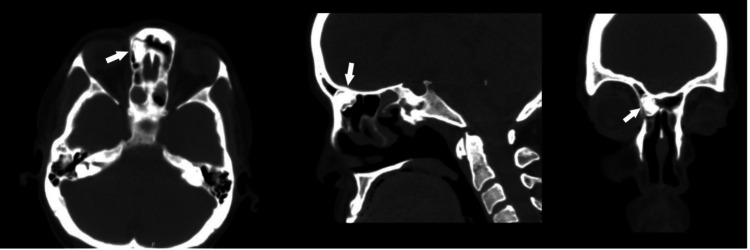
Preoperative CT scan Right frontal sinus osteoma (arrow) in axial, sagittal, and coronal views.
